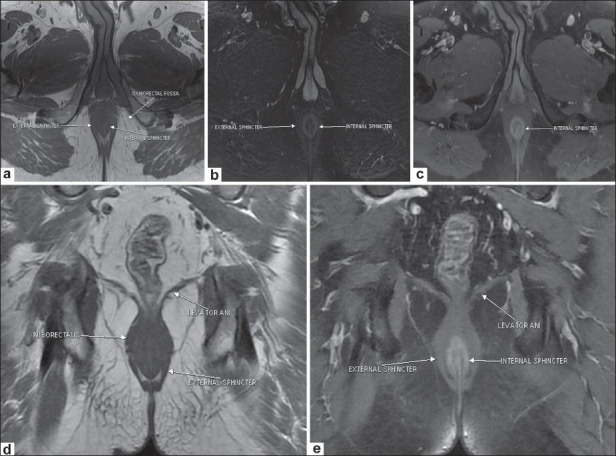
Normal MRI anatomy of the sphincters. Axial T1W image (a) shows the internal and external sphincters. Axial fat-saturated T2W image (b) shows the relatively hyperintense internal sphincter bounded laterally by the hypointense external sphincter. Postcontrast axial T1W image (c) depicts normal enhancement of the internal sphincter. Coronal T1W image (d) delineates the levator ani. Coronal postcontrast T1W image (e) shows the internal and external sphincters and the levator ani
